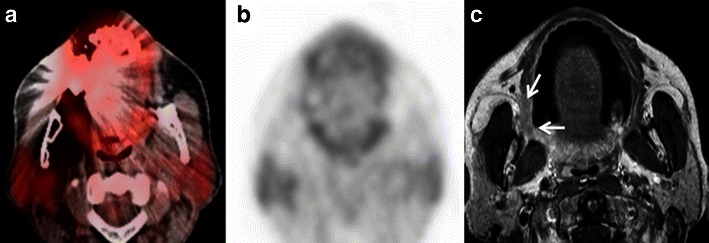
a Axial PET/CT image demonstrates extensive streak artefacts from right-sided dental implants in a patient with clinically proven SCCof the right retromolar trigone. The known lesion in the right retromolar trigone is completely obscured by the streak artefacts. b Corresponding axial FDG-PET image depicts no uptake in the region of the right retromolar trigone thereby yielding a false-negative result. c Corresponding axial contrast-enhanced T1W MR image detects the infiltrative mass in the right retromolar trigone (*white arrows*). The extent of the lesion as seen on MRI was afterwards confirmed surgically. MRI is less affected by dental artefacts as compared to CT
